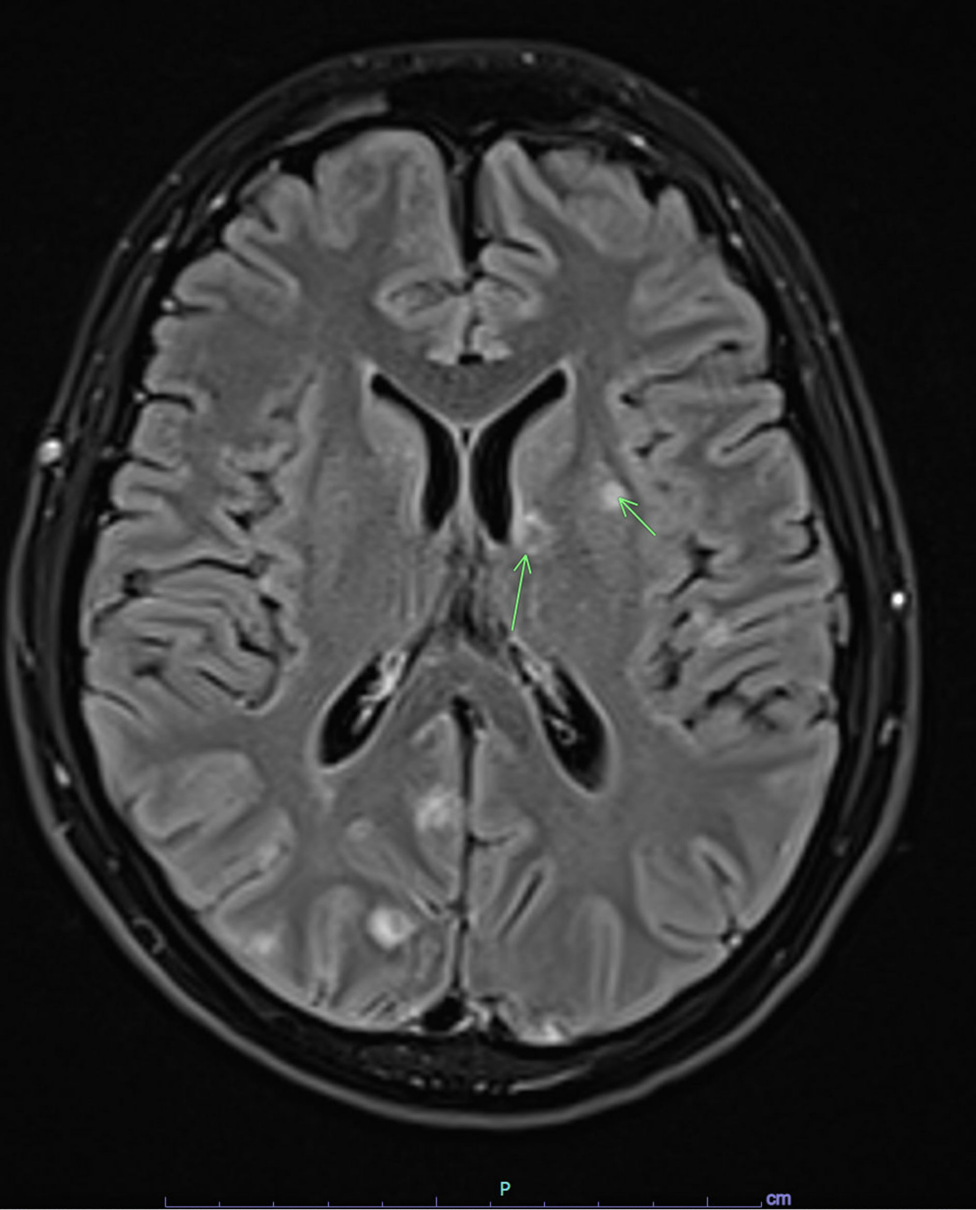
Magnetic resonance imaging of the brain with contrast demonstrating numerous small foci of enhancement at the brain surface within the sulci and throughout the bilateral cerebral hemispheres (arrows point to lesions).
